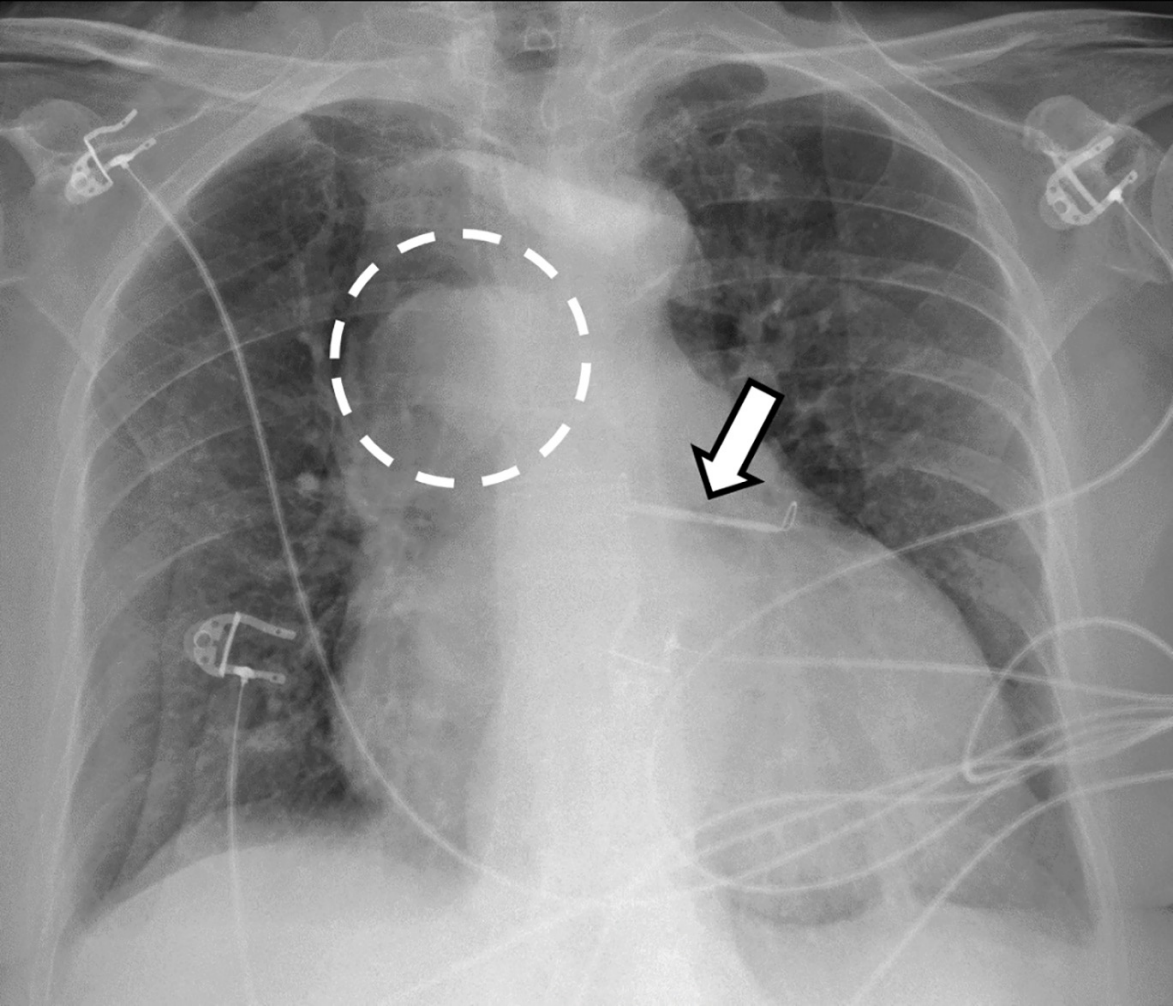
Chest radiograph showing cardiomegaly and a nodular right‑paratracheal opacity (dashed circle), suggestive of an enlarged azygos vein. A metallic opacity (arrow) from a previous clipping of the left atrial appendage is also visible.
